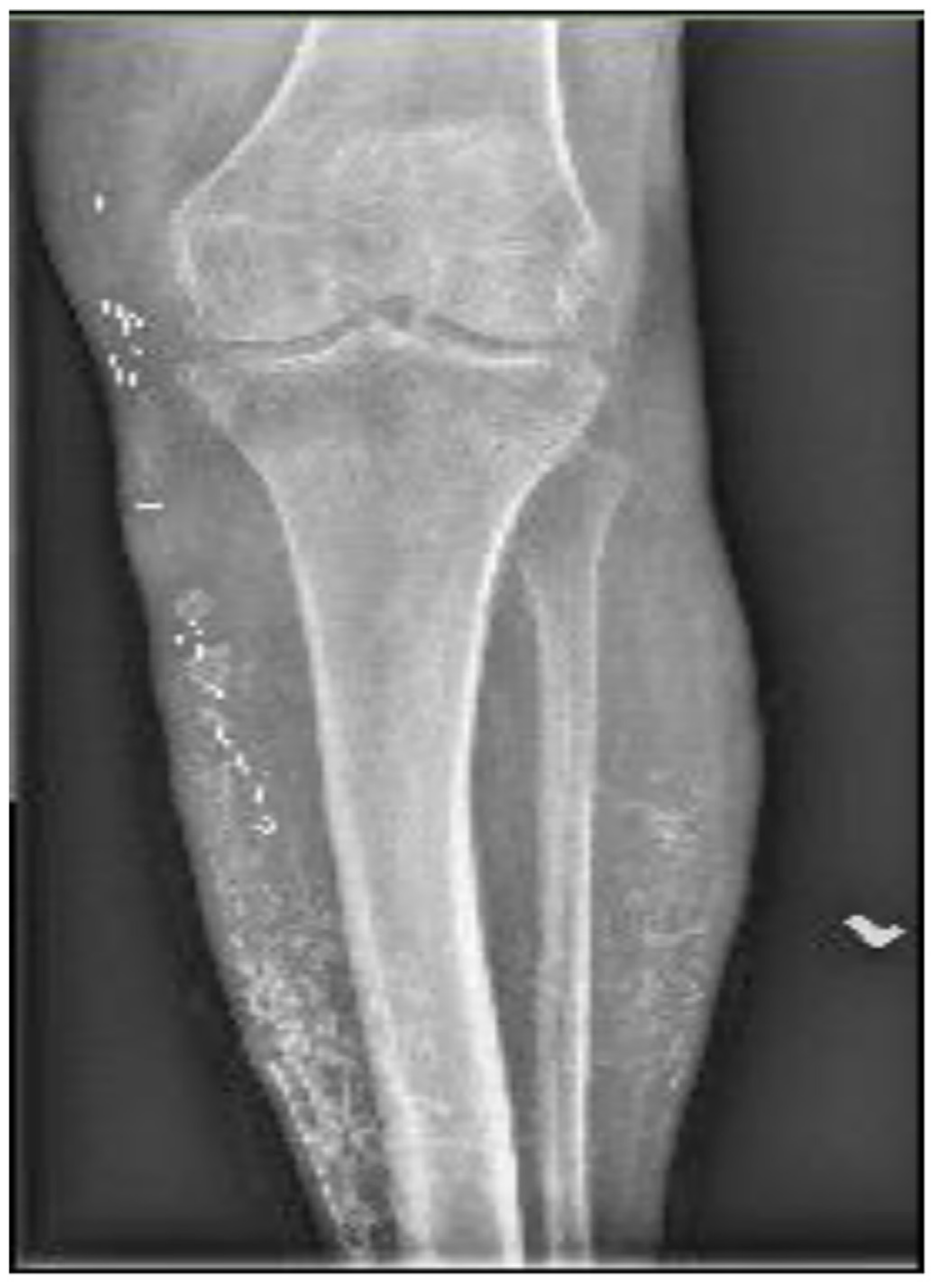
Plain radiograph showing long segments of calcifications, consistent with Heinz-Lippmann disease.
